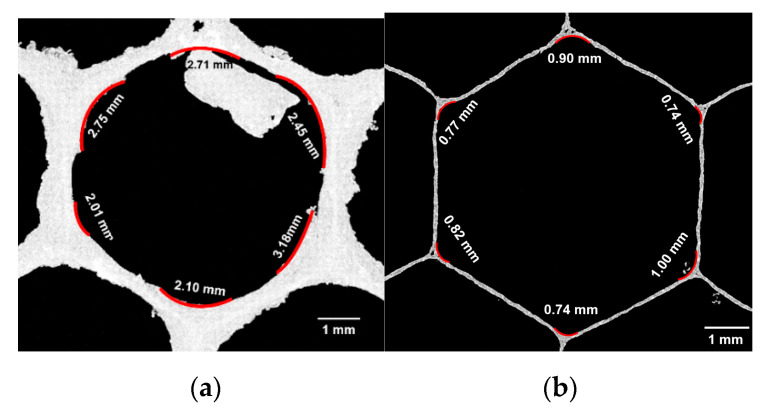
X-ray tomography of a single cell from natural honeycomb, showing (a) an average corner radius of 2.53 mm at the top of the coping, and (b) a radius of 0.96 mm in the interior of the cell.
